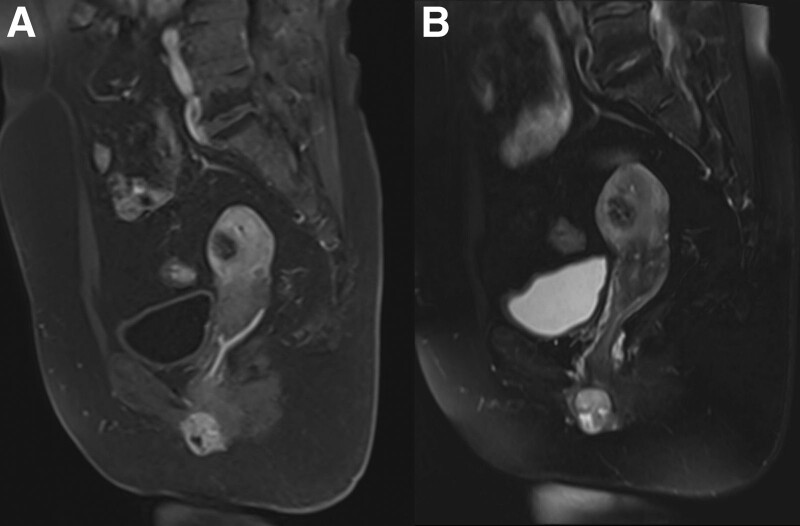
(A, B) Magnetic resonance imaging (MRI) showing that there was a circular signal anomaly on the left side of the vagina, with a clear boundary and high signal hybridity on T2W1.
